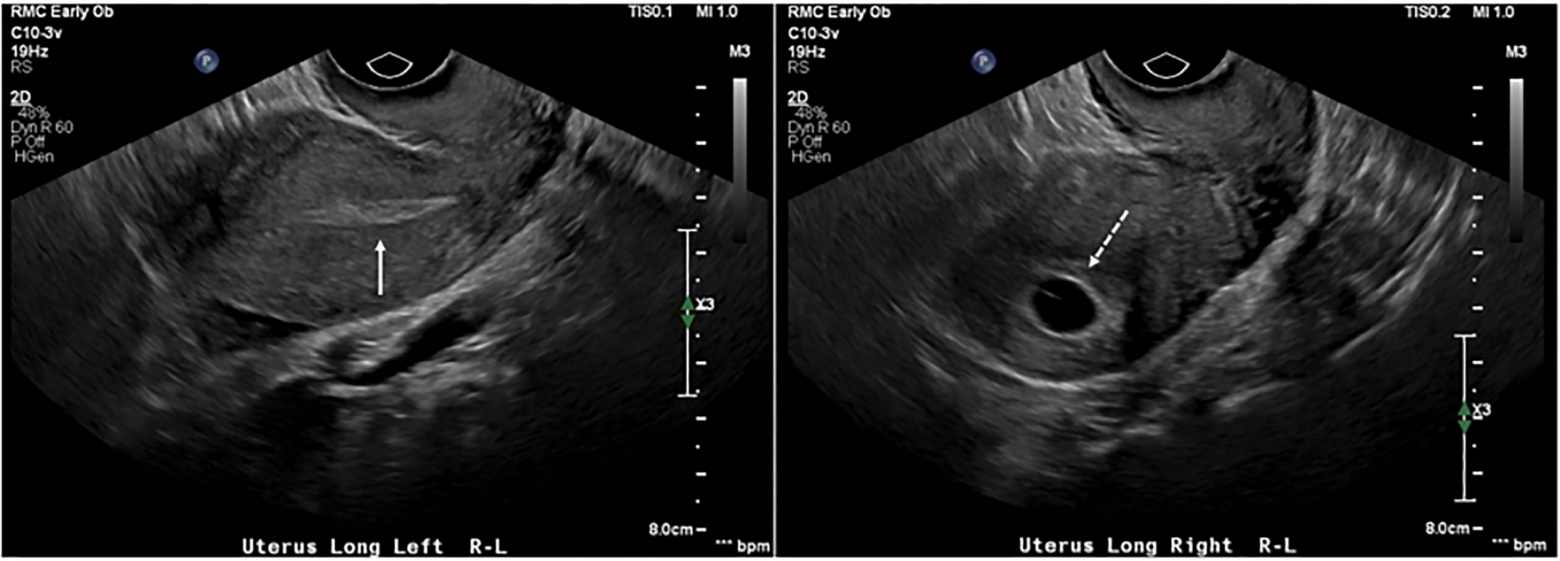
Transvaginal ultrasound. Longitudinal views of the uterus demonstrate uterus didelphys configuration with the absence of gestational sac in the left uterus (solid continuous arrow, left) and the presence of a gestational sac in the right uterus (interrupted arrow, right).
